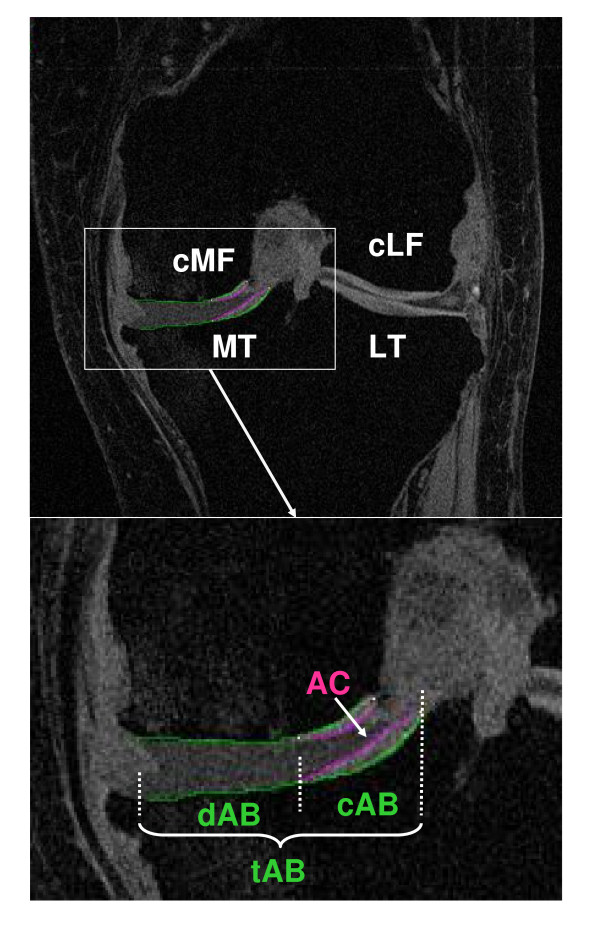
Baseline double oblique coronal MR image acquired using a fat suppressed SPGR sequence with a 1.5 mm slice thickness and 0.31 mm × 0.31 mm in plane resolution. The area of the cartilage surface (AC) and the total subchondral bone area (tAB) are manually segmented in the medial tibia (MT) and weight-bearing medial femur (cMF). The part of the tAB covered by AC is defined as the cartilaginous area of bone (cAB), that not covered by the AC as the denuded area of bone (dAB).
